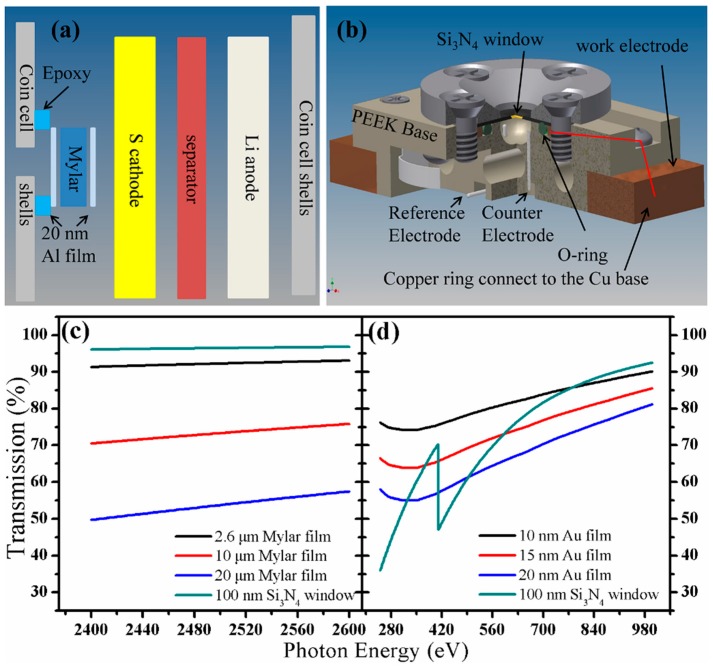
The scheme of (a) a modified coin cell and (b) a three-electrode electro-chemical cell (figure adapted from Reference [14]); and X-ray transmission at (c) soft X-ray energy region and (d) tender X-ray energy region through different materials.
